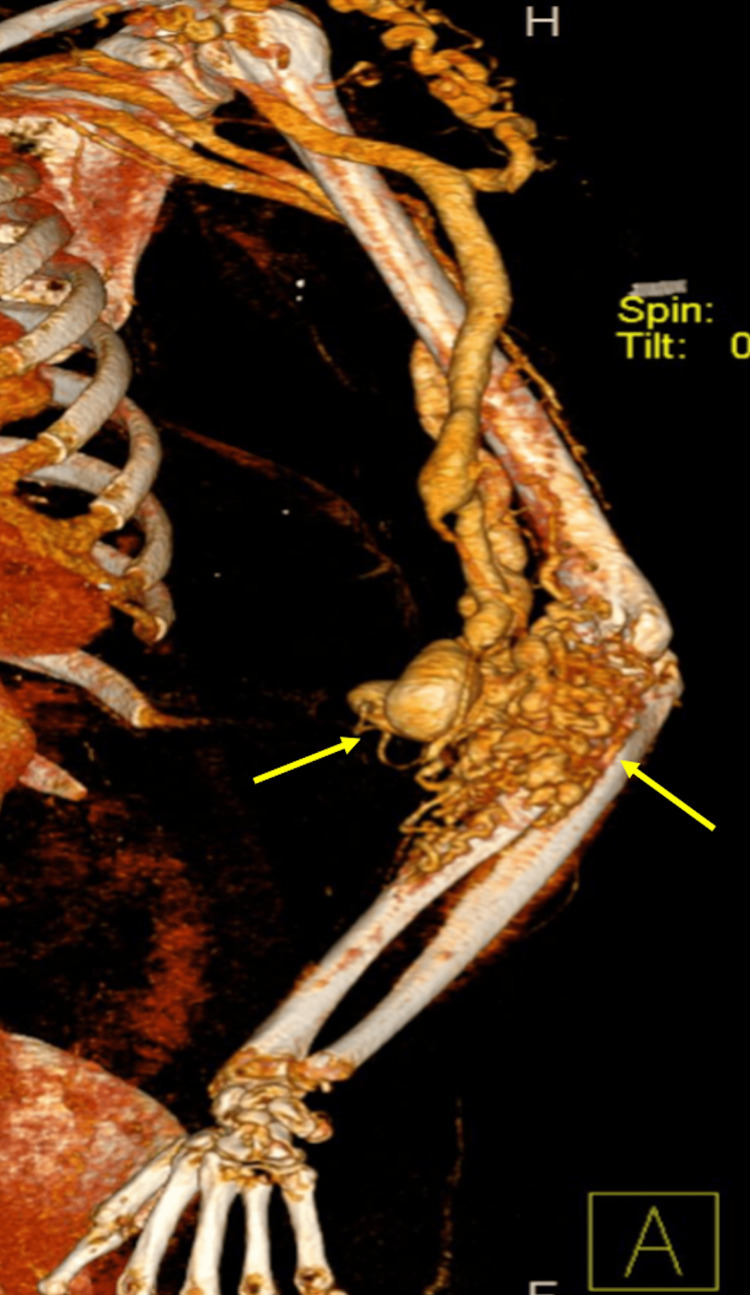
3-dimensional computerized tomography (CT) scan showing revealing tortuous arm and forearm vessels
